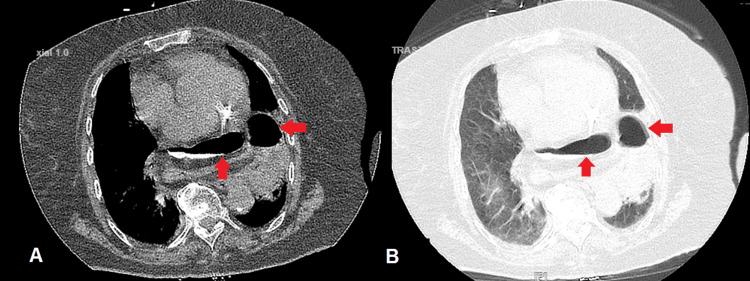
CT chest showing the hiatal hernia. A is the mediastinal window, B is the pulmonary window, and the arrows in both images point to part of the stomach and parts of the colon.
